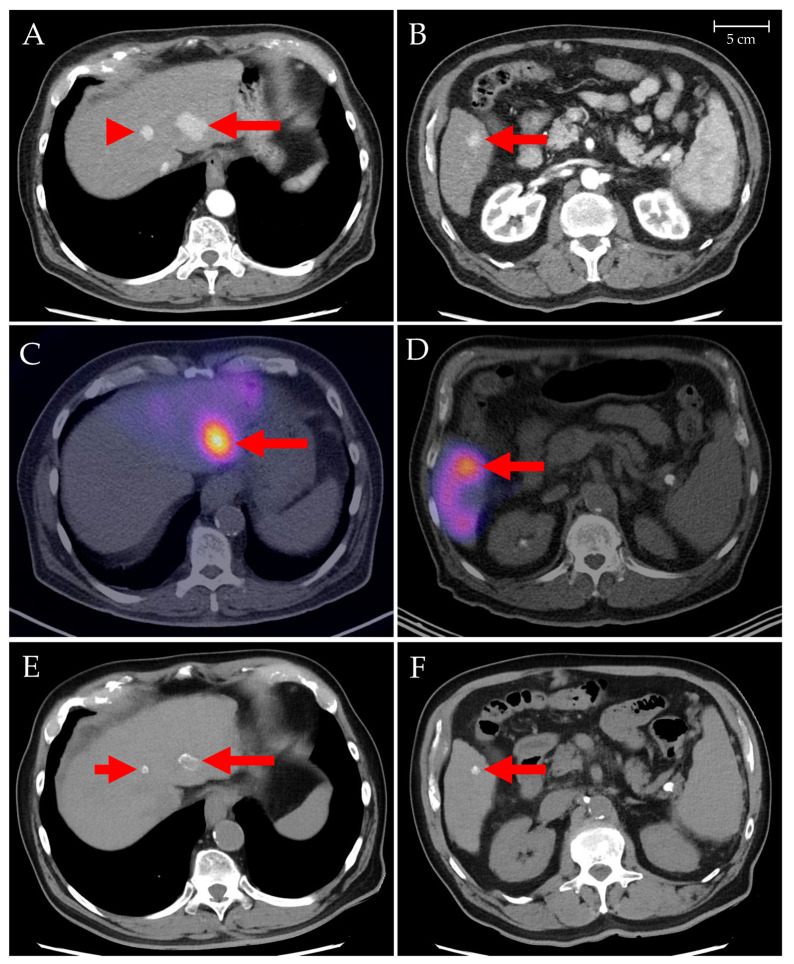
Patient #10. The contrast-enhanced CT showed multiple hypervascularized lesions in both liver lobes, the largest in segments II ((A), arrow) and V ((B), arrow). The small lesion was in segment IVa ((A), arrowhead). SPECT/CT fusion images after left-lobar (C) and right-lobar (D) ^166^Ho-TARE procedures show distinct microsphere accumulations in the lesions (arrows). Follow-up CT after 3 months revealed complete tumor remission, with lesions decreased in size and without contrast enhancement. Irregular hyperdensities, corresponding to the microsphere accumulations, were noted on non-contrast CT ((E,F), arrows).
